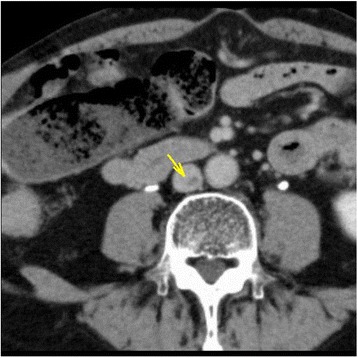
Contrast-enhanced computed tomography at 7 days after the initiation of anticoagulation therapy. The yellow arrow indicates reduced thrombosis
